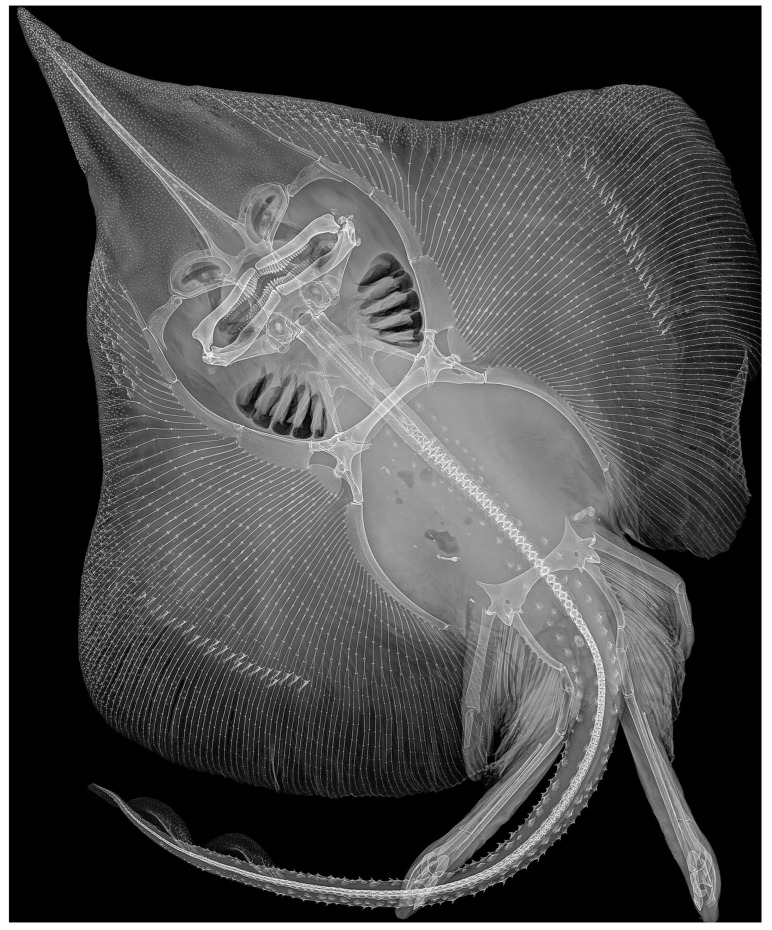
Leucoraja longirostris* n. sp., ZMH 26260, adult male holotype, 711 mm TL, radiograph in total dorsal view.
